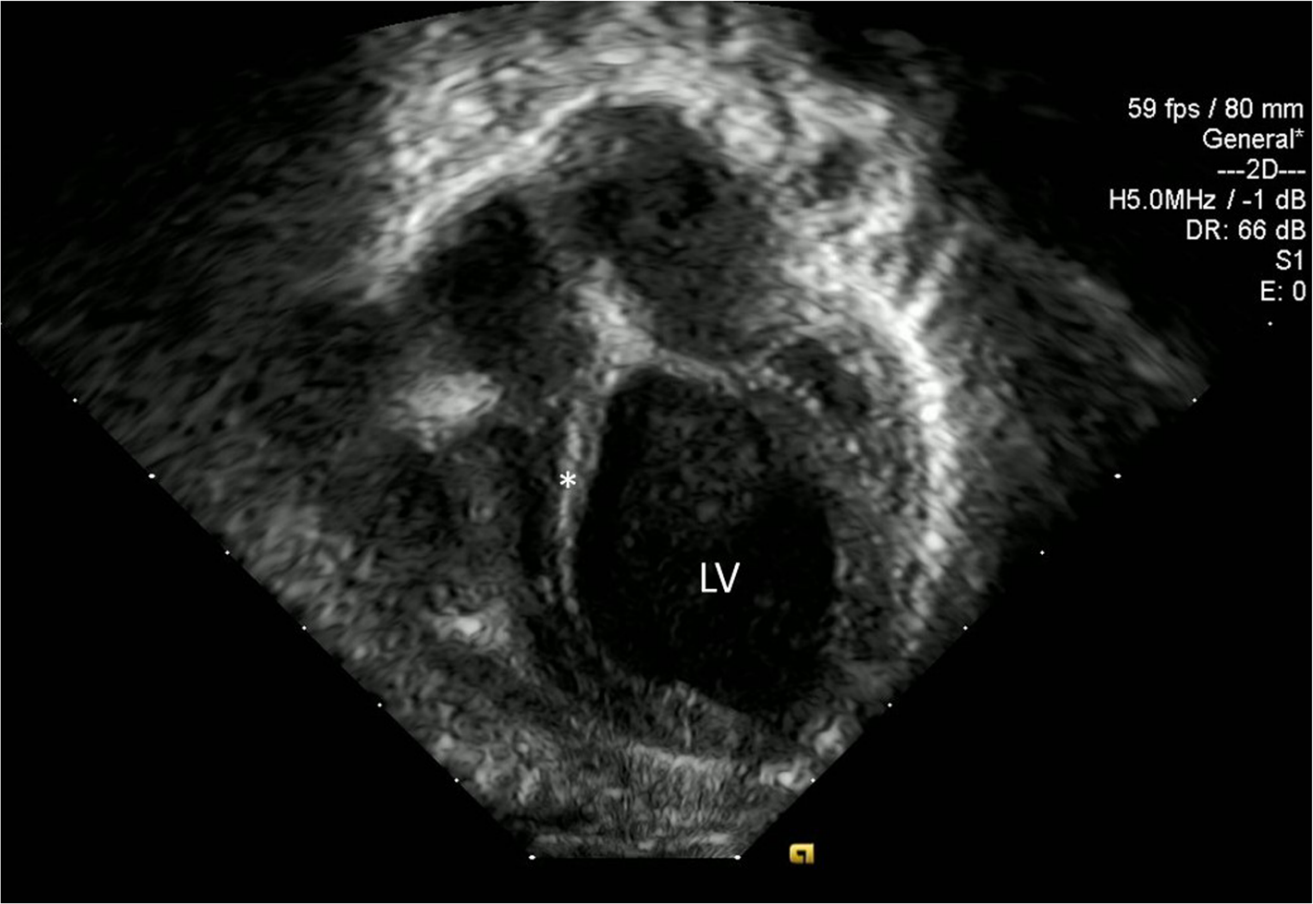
Echocardiography at 5 weeks of life showing severely dilated left ventricle (LV) and thin echogenic, dyskinetic ventricular septum (*). The origins of coronary arteries were normal with an ejection fraction of 25%, mild tricuspid insufficiency, and mild mitral insufficiency
